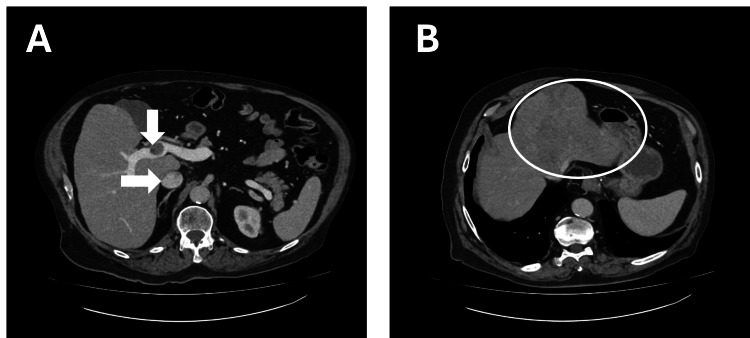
CT imaging demonstrated features of a cirrhotic liver with a large mass in the left lobe, with tumor infiltration into the portal vein, inferior vena cava (IVC), and right atrium. (A) Vascular tumor invasion of the portal vein (left arrow) and the inferior vena cava (downward arrow). (B) Left hepatic mass extending into the portal vein and inferior vena cava.
